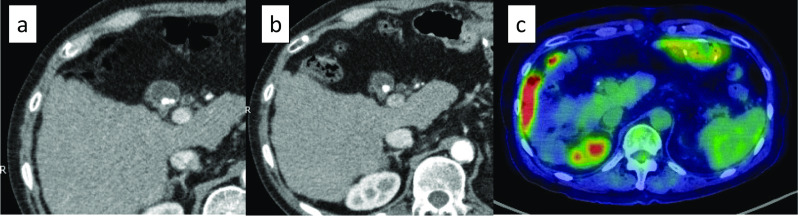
a CT findings 2 years before the second gastric surgery. b Preoperative CT findings. It was difficult to identify the primary site, and there were no findings suggestive of distant or LN metastasis. There were no abnormal findings in the gallbladder, except for the presence of gallstones, and there were no changes over time. c FDG-PET/CT showing negative uptake in the stomach, LNs, and gallbladder
